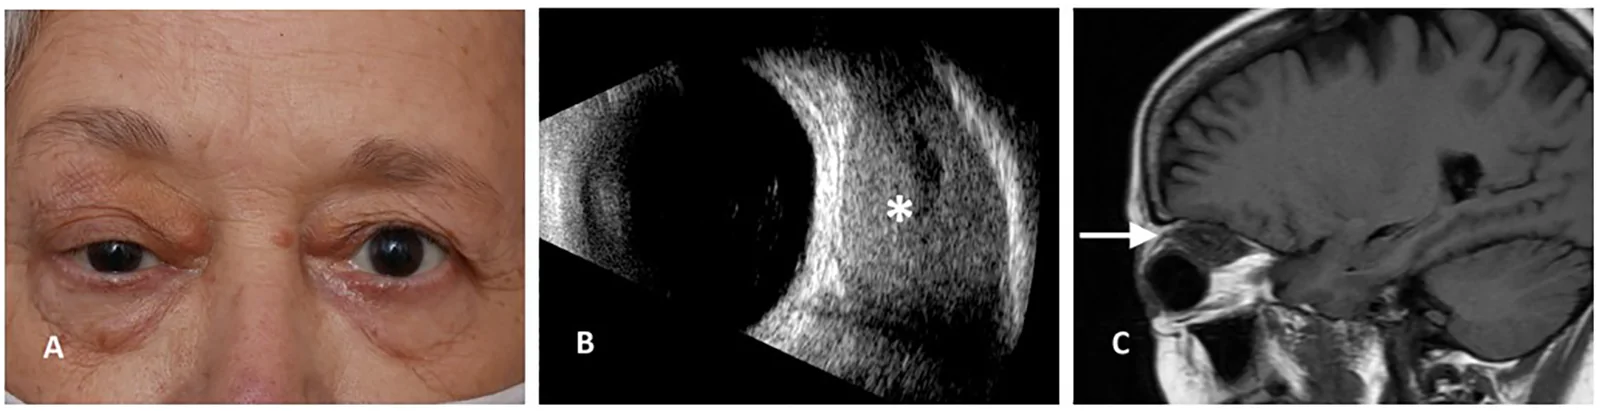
Orbital Metastasis. (A) External photo of a 64-year-old female presenting with proptosis, restricted eye motility, inferior displacement of the right eye, and a history of cutaneous melanoma 22 years ago. (B) Longitudinal B-scan aimed superiorly shows a massive, fusiform, homogeneous, low reflective enlargement of the superior rectus (asterisk). (C) Sagittal T1-weighted MRI shows a bulging mass of the superior rectus muscle belly, with homogenous signal intensity (arrow). The asterisk (*) is used to label an area of the figure.
